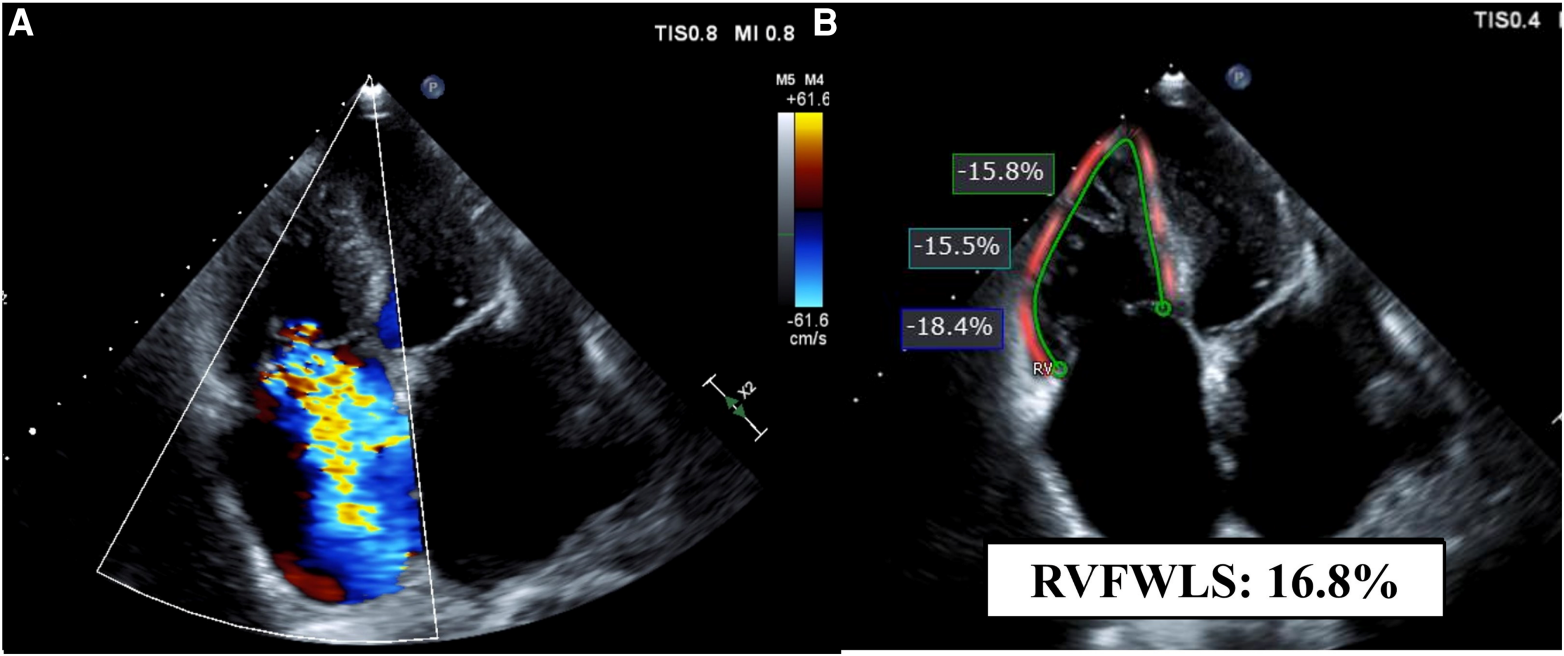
A representative case. Echocardiographic images of a patient with severe tricuspid regurgitation and atrial fibrillation on the RV-focused apical four-chamber view. (A) Color Doppler echocardiogram shows severe tricuspid regurgitation. (B) Measurement of RVFWLS. FWLS, free wall longitudinal strain; RV, right ventricular.
